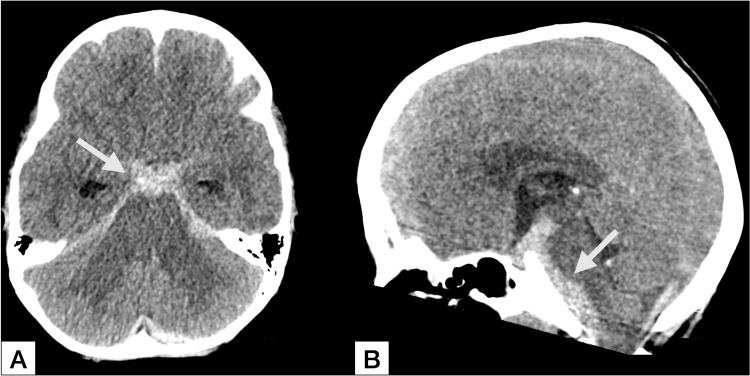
Initial non-contrast computed tomography imaging Axial (A) and sagittal (B) non-contrast head computed tomography imaging demonstrating diffuse subarachnoid hemorrhage, with predominant involvement of the basal cisterns in (A) and the posterior fossa in (B) (arrows).
